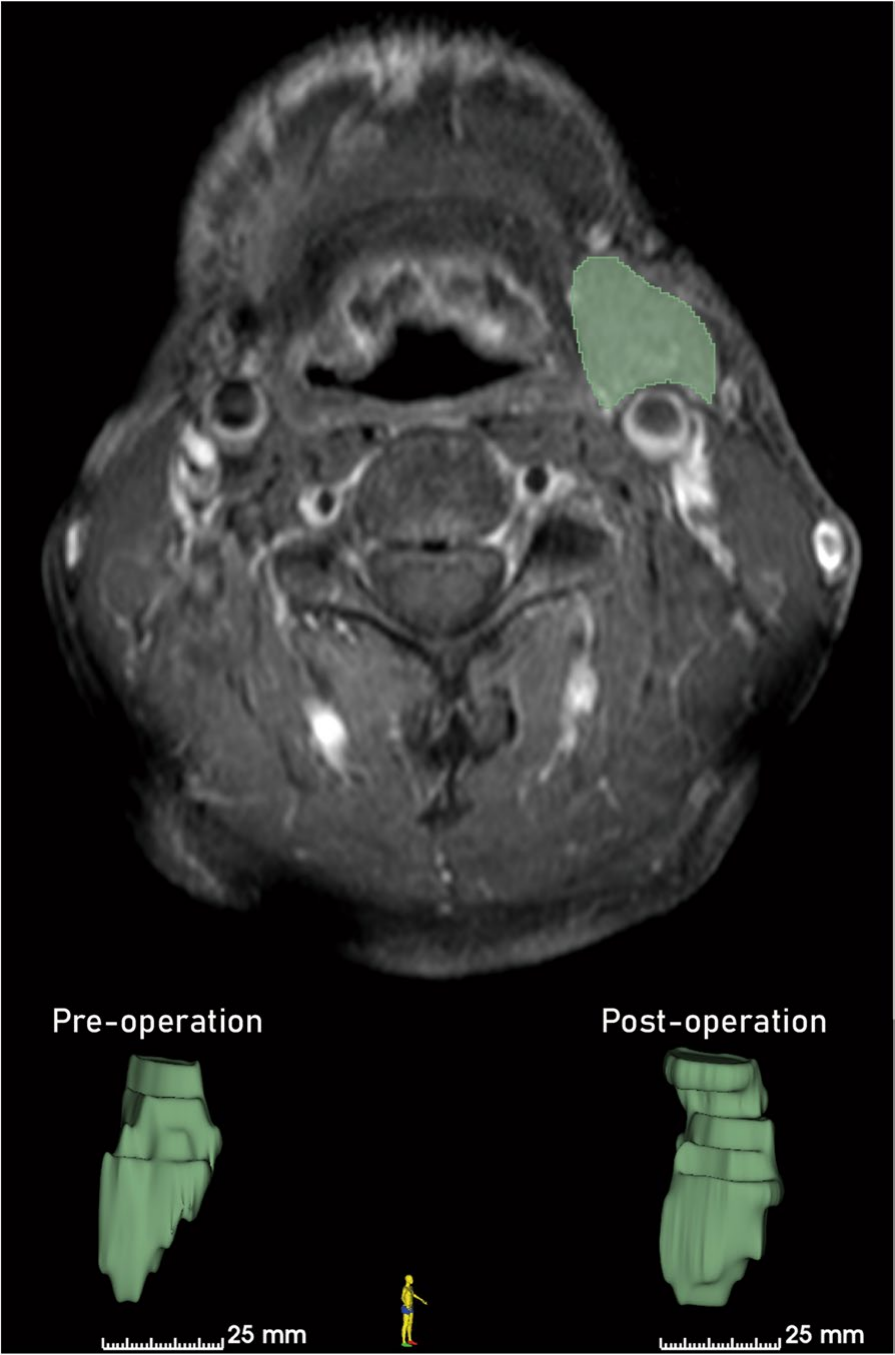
Volumetric analysis of the contralateral submandibular gland using 3D Slicer software (version 5.6.2). The volume measurements were performed on preoperative, immediate post-operative, and follow-up 3D radiographic data. The segmentation of the submandibular gland was manually delineated slice by slice (upper image), and the software calculated the total gland volume based on these segmentations (lower left and right images)
